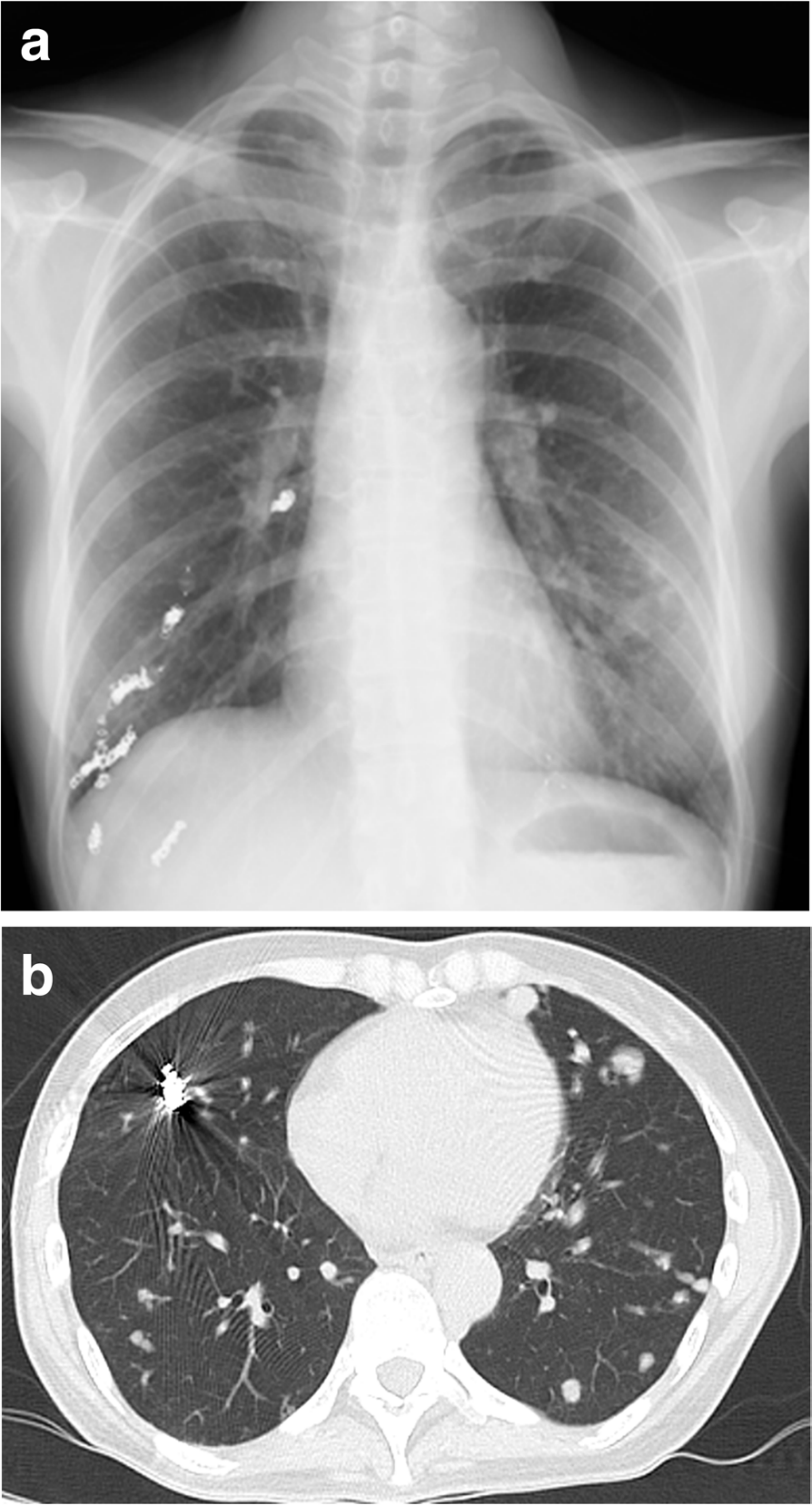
a Chest radiograph. The chest radiograph shows preoperative coil embolization of the pulmonary arteriovenous malformations on the right lung. b Computed tomography of the chest. The computed tomography of the chest shows bilateral pulmonary arteriovenous malformations
